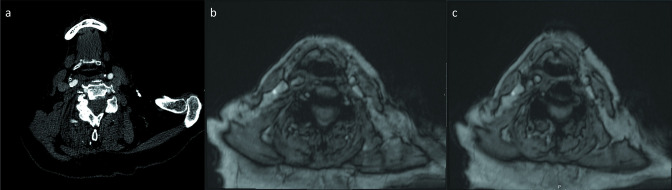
(a) CTA of the neck demonstrates atherosclerotic calcification in the right carotid bulb. (b) On MRA of the neck, it is more difficult to see these right carotid bulb calcifications. (c) Slightly more inferior MRA neck image shows hypointense signal along the surface of the bilateral common carotid arteries due to presence of intravascular ferumoxytol. CTA, CT angiography; MRA, MR angiography.
